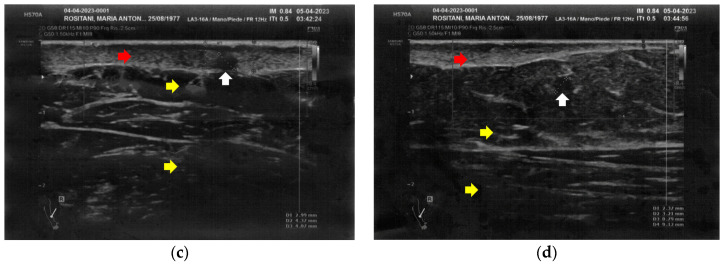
Ultrasonography of the forearms at T0. (a) Cicatricial fibrosis (red arrow) of the left forearm involves the whole dermal layer; (b) The inflammation (yellow arrows) is extended down to the deeper layer and, in particular, it involves the surgical grafts (green arrows); (c) Cicatricial fibrosis (red arrow) is also evident on the right side, where the adipose tissue appears inhomogeneous (yellow arrows) and where a cicatricial granuloma is present (white arrow); (d) The aspect described in Figure 2c is similar in other areas of the right forearm, where other cicatricial granulomas are present (white arrow).
